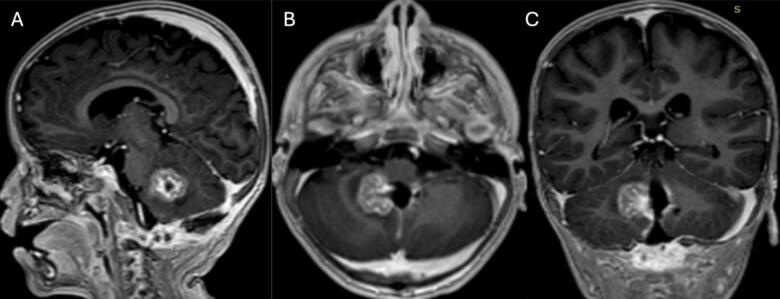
Magnetic resonance imaging of the brain: Second MRI after the First craniotomy showing an increase in the size of the enhancing residual lesion.
